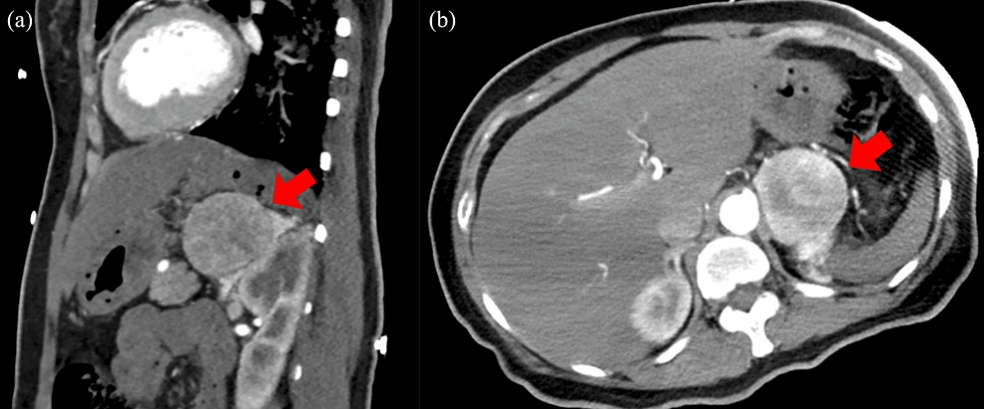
Contrast-enhanced CT scan performed at a previous hospital Physical findings of the patient. A 52-mm mass contiguous with the left adrenal gland is seen. (a) Sagittal section and (b) horizontal section.
